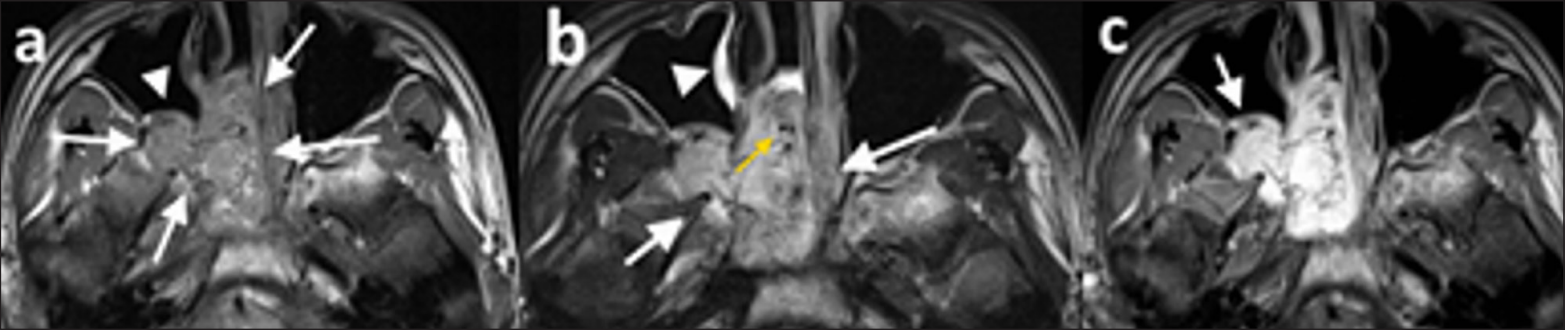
15-year-old male patient (patient 1), (a) axial T1-weighted precontrast, (b) axial T2-weighted precontrast, and (c) axial T1-weighted postcontrast MR images demonstrate a right-sided nasopharyngeal mass which enlarges the ipsilateral pterygopalatine fossa (*a and b white arrows*). The mass is isointense with the muscle on T1-weighted image (a) and hyperintense on T2-weighted image (b) and exhibits significant contrast enhancement (c). The posterior wall of the maxillary sinus demonstrates anterior bowing with resultant Holmann Miller sign (*a, white arrowhead; c, arrow*). The tumor demonstrates signal-void regions (*b, yellow arrow*) and inflammatory signal changes in maxillary sinuses (*b, white arrowhead*).
